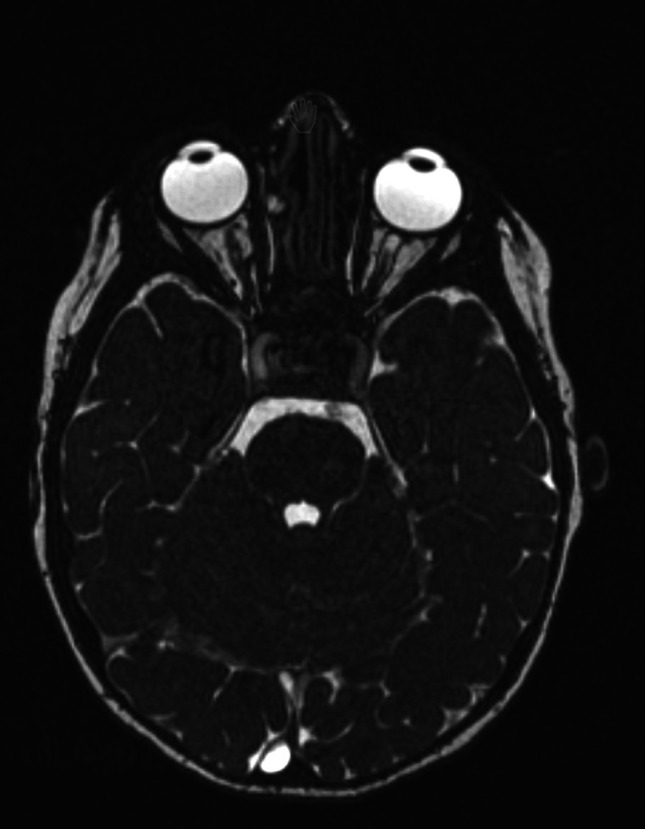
T2 weighted MRI scan in axial plane ten months after surgery
